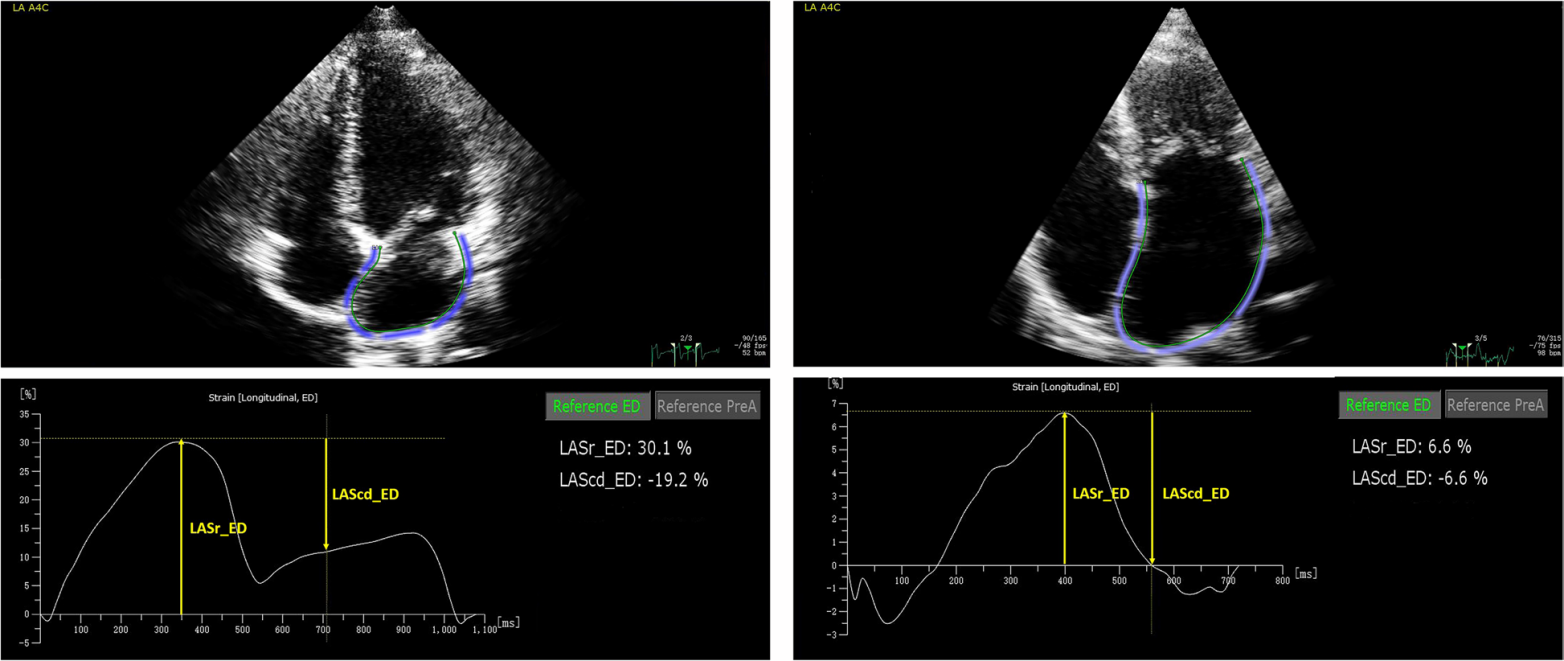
The figure presents the strain of the left atrium using two-dimensional speckle-tracking echocardiography in patients with sinus rhythm (A, B) and atrial fibrillation (C, D). AutoStrain LA software will automatically detect and track the contours and then advance to the analysis step. Left atrial reservoir strain and left atrial conduit strain are displayed based on the end-diastolic option
